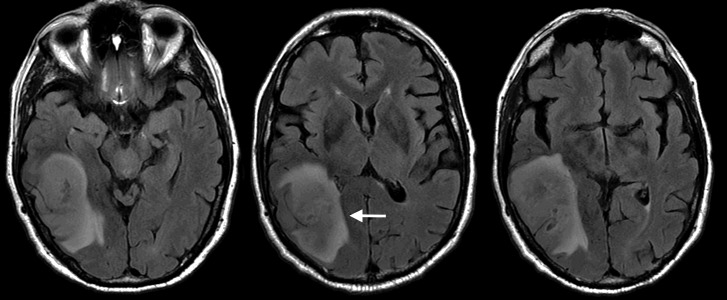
Axial T2 FLAIR MRI Minimal mass effect and fluid-attenuated inversion recovery (FLAIR) hyperintensity relative to the size of the mass (arrow) MRI: magnetic resonance imaging
